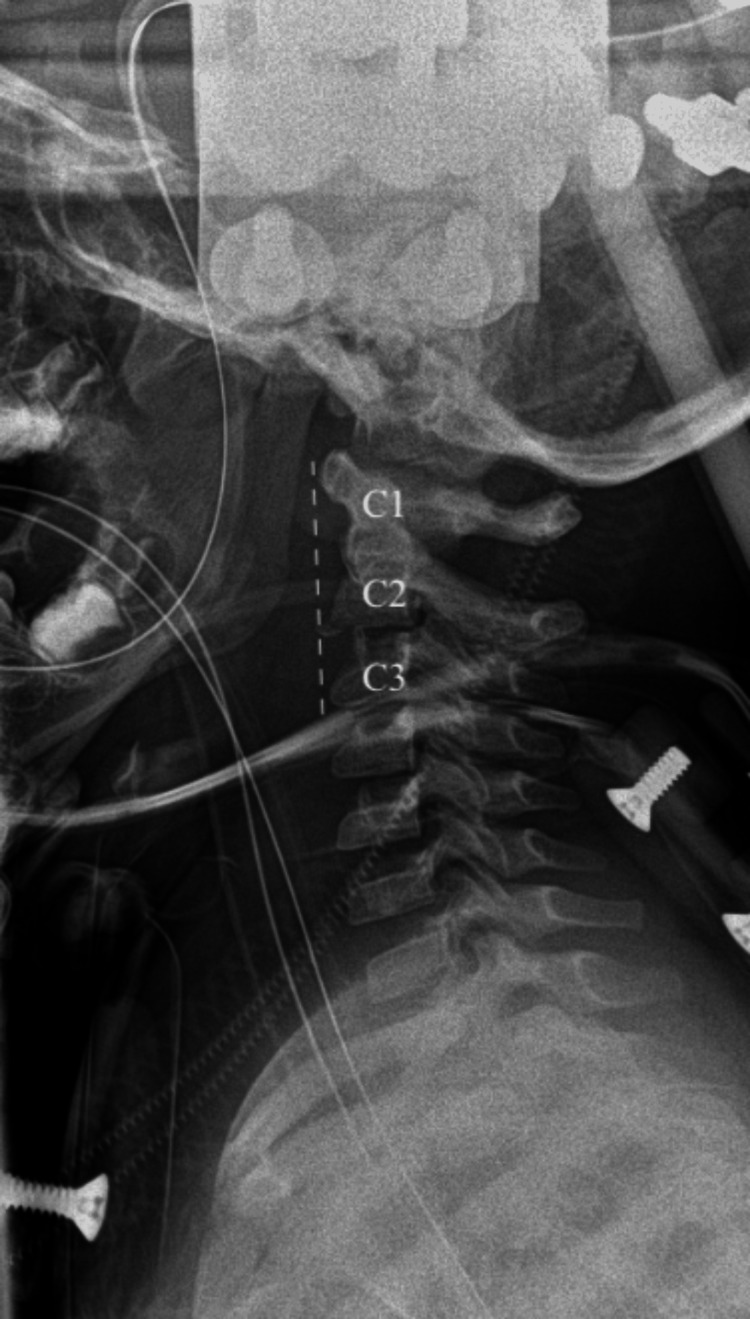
Lateral cervical spine X-ray from hospital day 1 following revision of halo device in the operating room demonstrating more satisfactory alignment of cervical spine when compared to Figure 6
